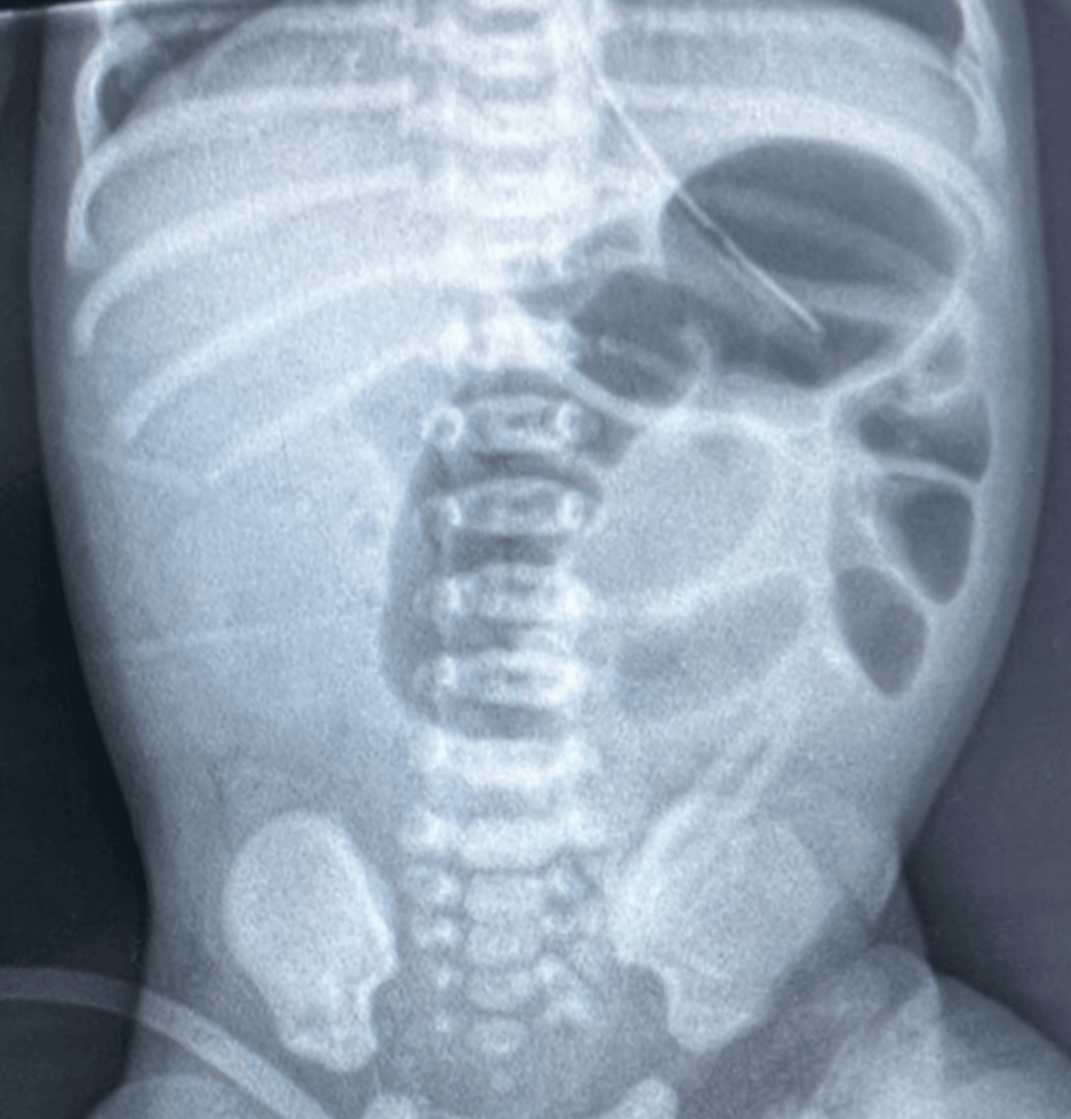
Plain abdominal radiography showing dilated small bowels.
